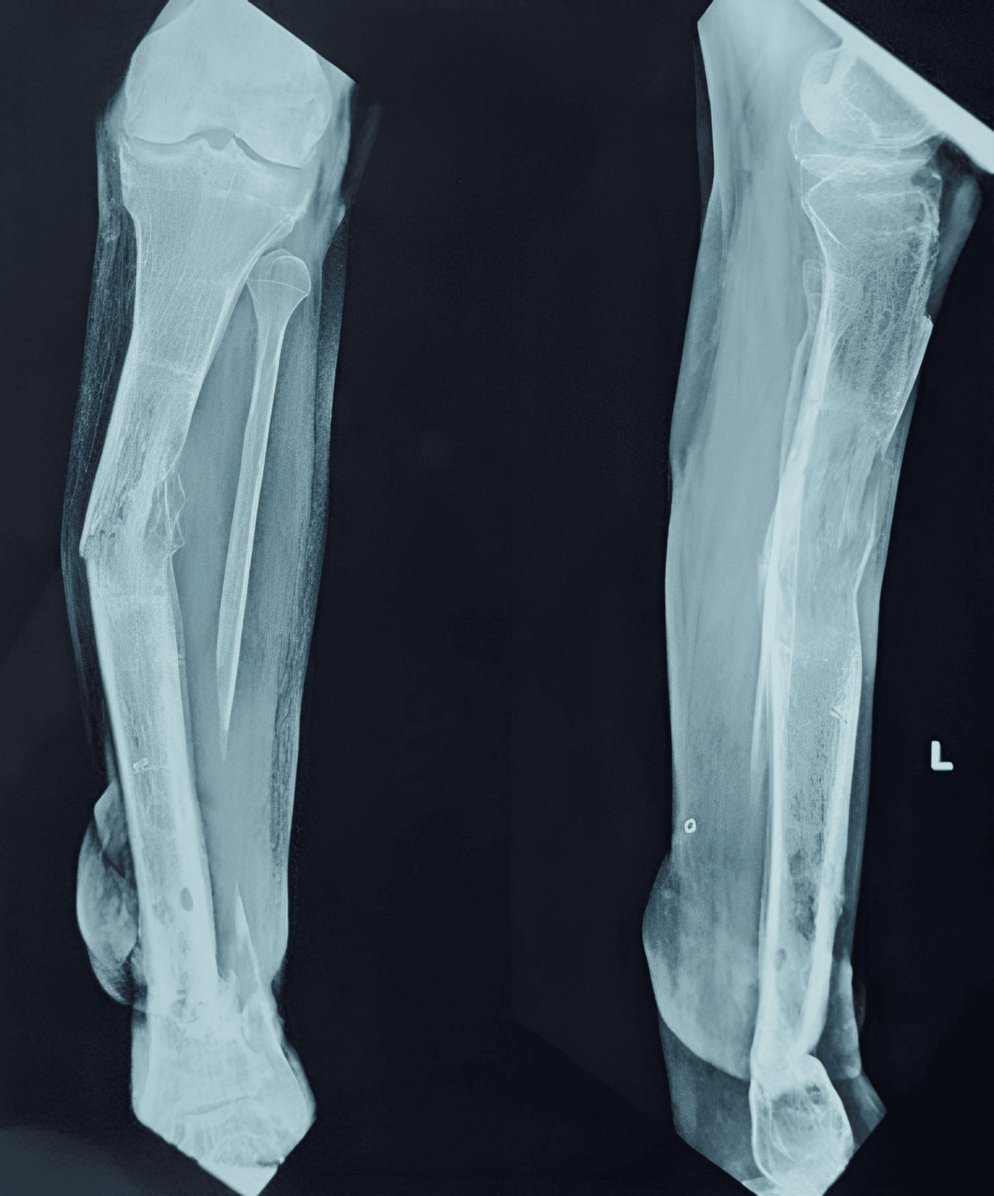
X-ray showing the fracture at the distal end of the distraction site
